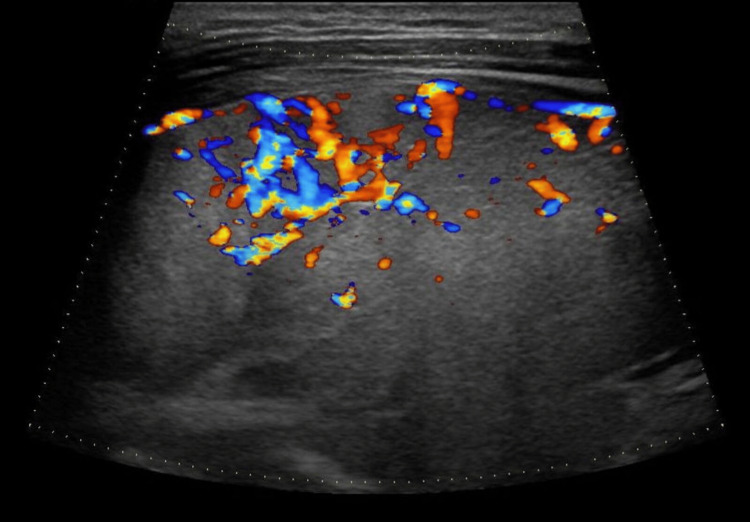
Color Doppler ultrasound demonstrating diffuse hypervascularity of the thyroid gland (“thyroid inferno”) characteristic of Graves’ disease.
